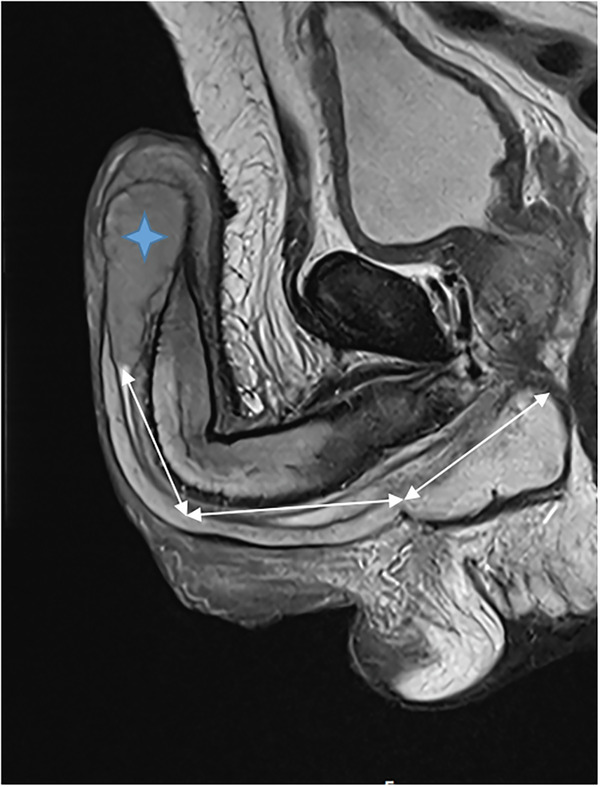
The length of healthy proximal penile tissue, measured from the proximal margin of the tumor to the penoscrotal junction on sagittal T2-weighted images, was here greater than 3 cm. Penile tumor (star)
